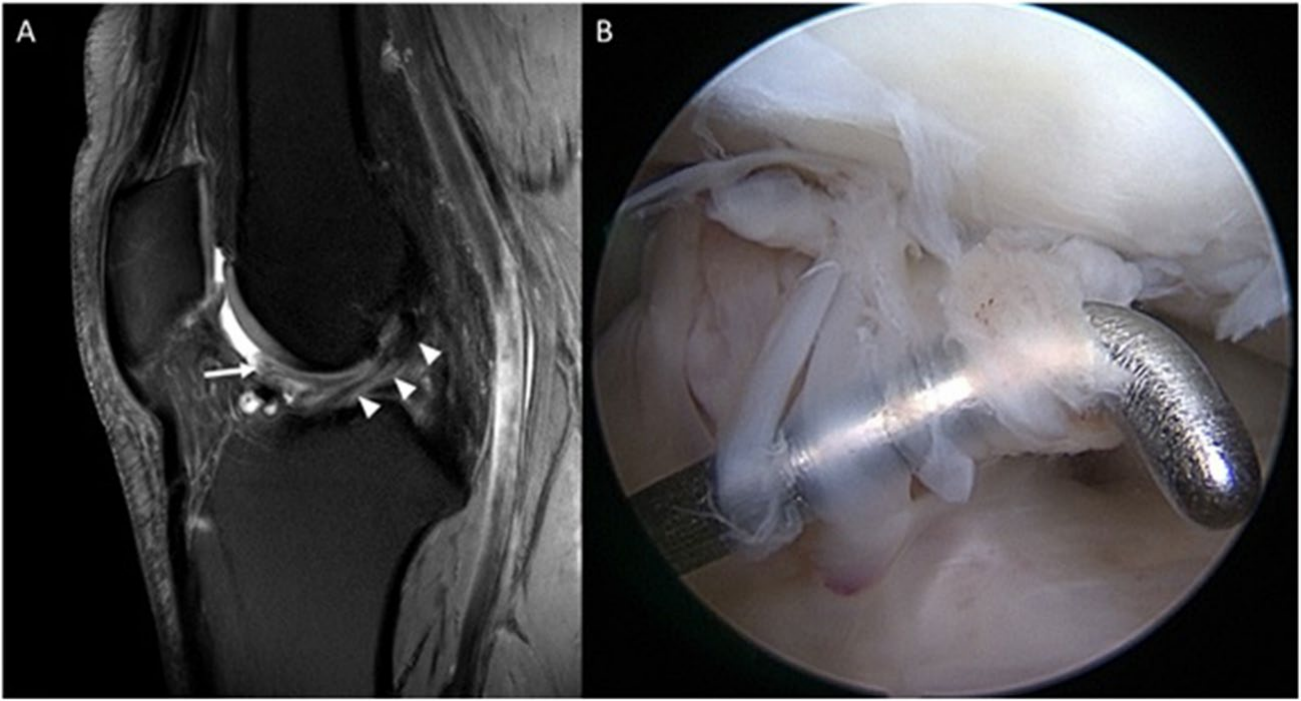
A 7T proton density-weighted fat-saturated MRI image of a 24-year-old male patient 1.5 years after primary ACL repair with fraying of the ACL and prolapse of ACL fibers into the intercondylar notch (arrow). The remaining parts of the ACL appear continuous (arrowheads). B Corresponding arthroscopic appearance of fraying in the same patient. The repaired ACL itself was continuous and stable
